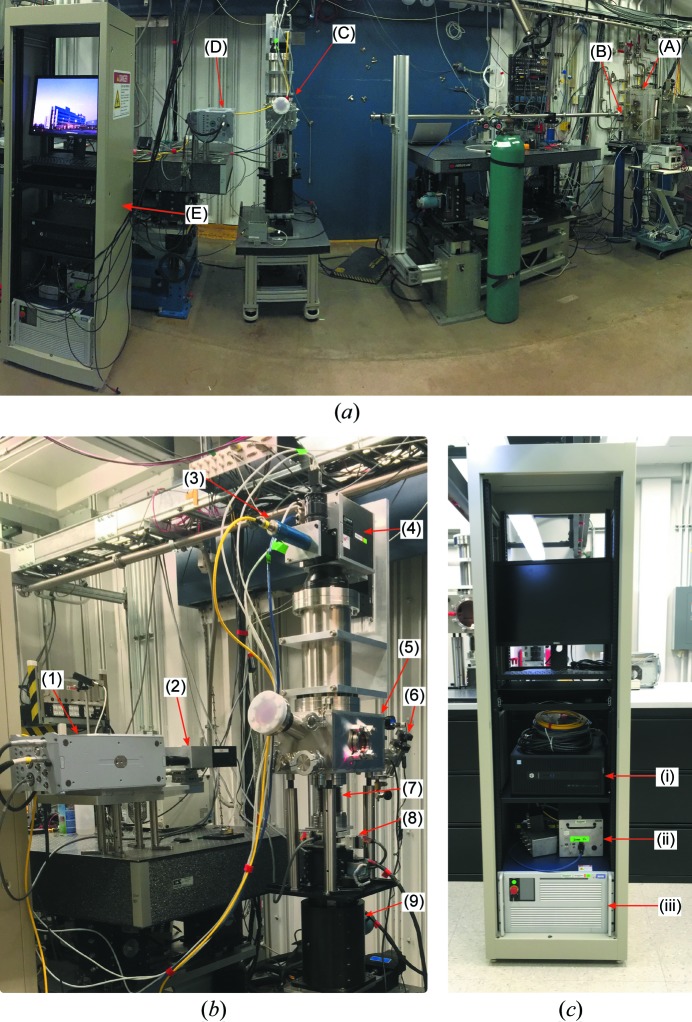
Photographs of the experimental setup. (*a*) Overview of the 32-ID-B beamline at the APS. (A) Slow shutter, (B) fast shutter, (C) laser AM experimental setup, (D) high-speed X-ray imaging setup, and (E) laser system control computer and laser source rack. (*b*) Detailed view of the setup. (1) High-speed camera, (2) scintillator–mirror–objective lens assembly box, (3) laser feeding fiber and collimator, (4) laser scanner, (5) experimental chamber, (6) connection for the vacuum pump, (7) vacuum-compatible bellows, (8) three-axis translational stages for sample manipulation, and (9) two-axis stage for laser–X-ray alignment. (*c*) A detailed view of the control rack. (i) Scanner control computer, (ii) power sources and connection boxes for the scanner, and (iii) laser source.
